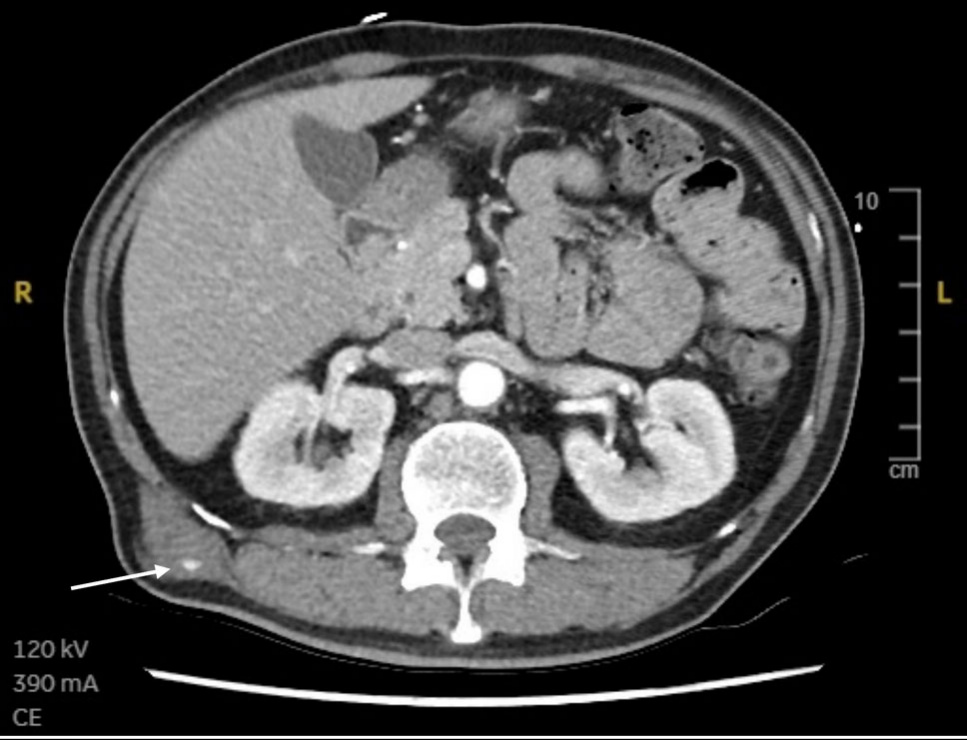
40-year-old male presenting to the emergency department after a motor bike accident. CT scan demonstrated a right latissimus dorsi intramuscular haematoma with contrast extravasation (arrow). The patient was successfully managed conservatively
